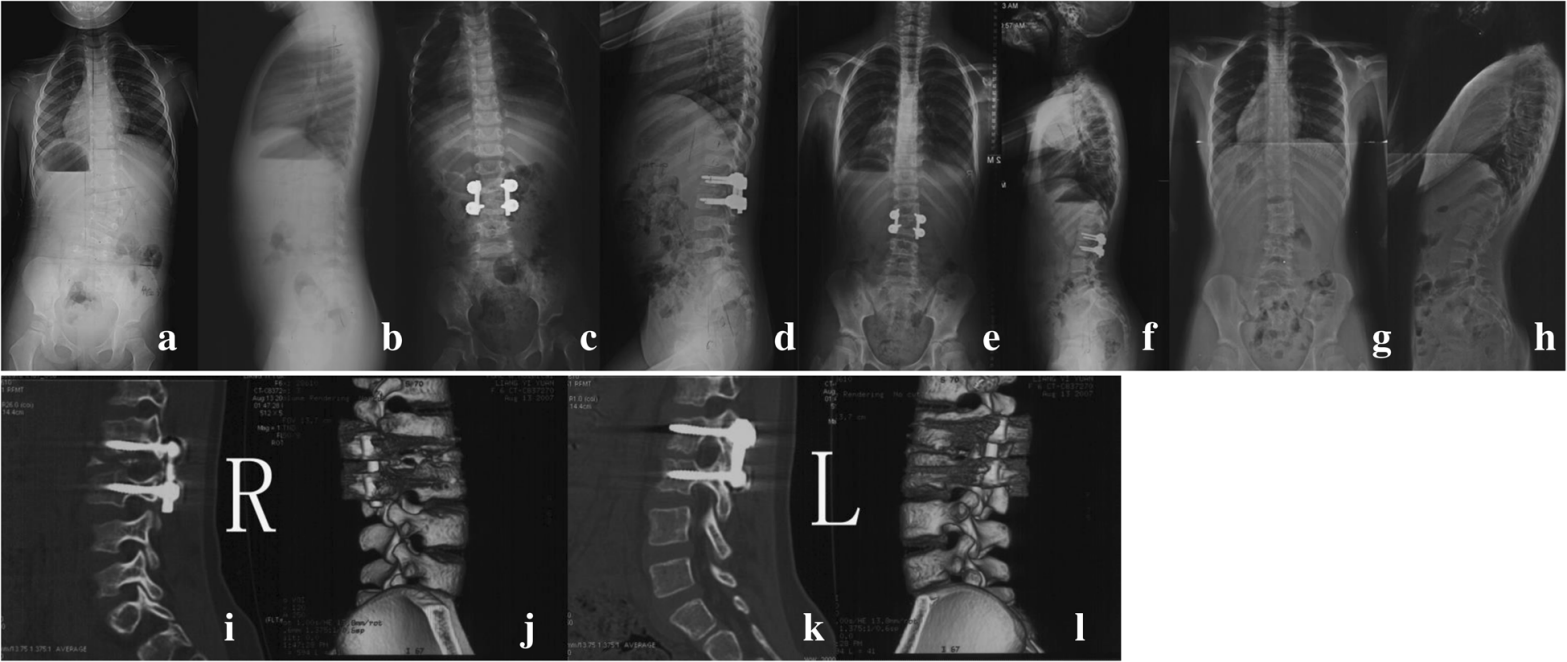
A 3-year-old girl with congenital scoliosis. Pre-operative radiographs (a, b) showed semi-segmented hemivertebra of L2. Post-operative radiographs (c, d) showed excellent correction by L2 hemivertebra resection. Radiographs at 7 years follow-up (e, f) showed L1 pedicles elongation and right pedicle screw dislodgement. CT scan and 3D reconstruction showed solid fusion at the fused segments was achieved and right pedicle screws dislodgement (i, j, k, l), and implants was removed (g, h)
